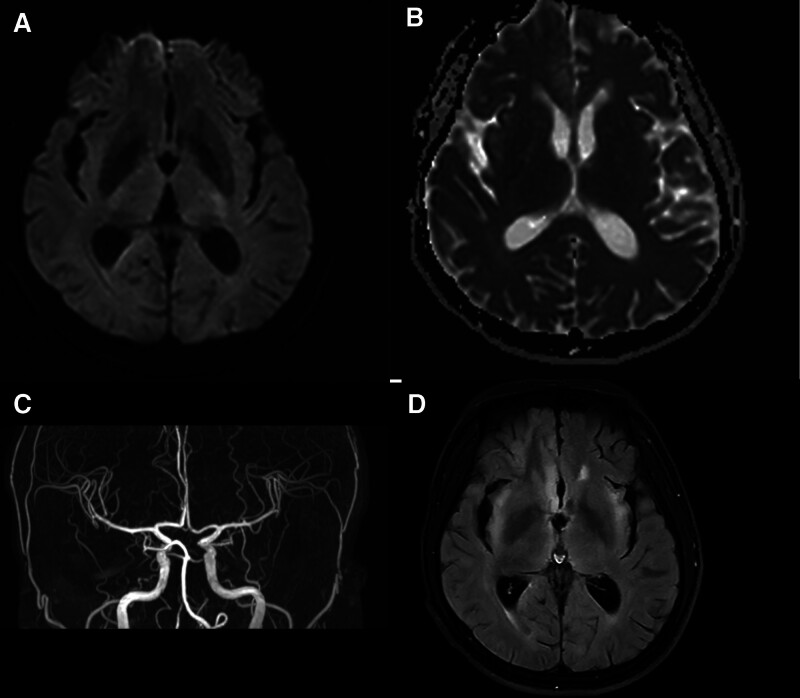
Head MRI findings on the third day of illness. The patient’s head DWI (A) and ADC (B) results were not abnormal and did not support the diagnosis of acute ischemic stroke. MRA of the head showed no intracranial vascular stenosis or occlusion (C). Multiple flaky abnormal signal images are seen on T2 Flair in the bilateral insula, hippocampal region, and lateral paraventricular (D). ADC = apparent diffusion coefficient, DWI = diffusion-weighted imaging, MRI = magnetic resource imaging.
